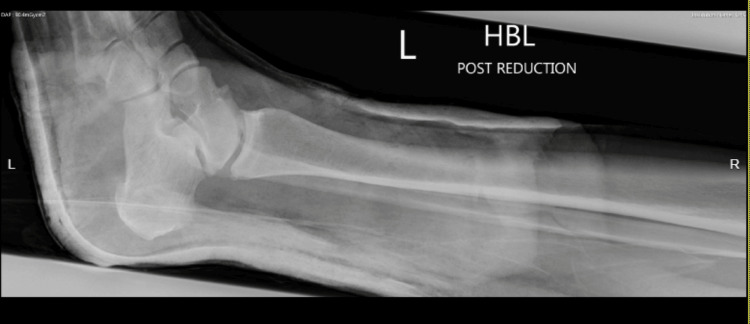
Lateral left ankle radiograph post-reduction under conscious sedation and application of a backslab
